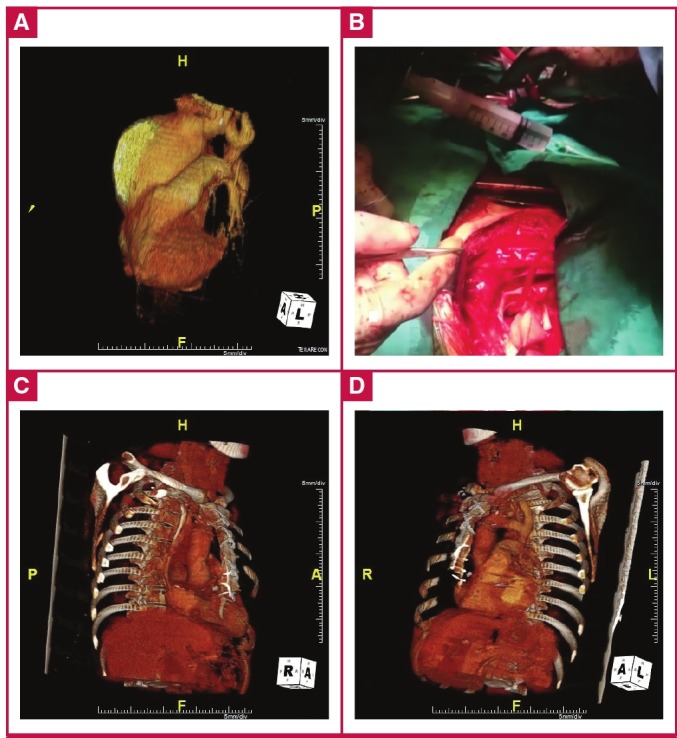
Pre-operative (A) and postoperative (C, D) threedimensional reconstructions from CT scans, and intraoperative (B) view of the aortic aneurysm.
